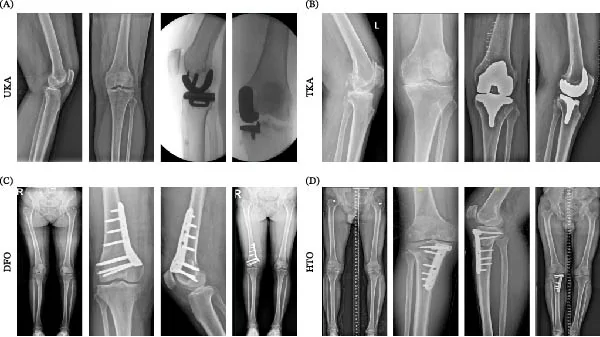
Radiographic presentations of various surgical approaches for OA. (A) Unicompartmental knee arthroplasty (UKA); (B) total knee arthroplasty (TKA); (C) distal femoral osteotomy (DFO); and (D) high tibial osteotomy (HTO). All radiographic images were obtained from the Orthopedic Medical Center, Linyi People’s Hospital.
